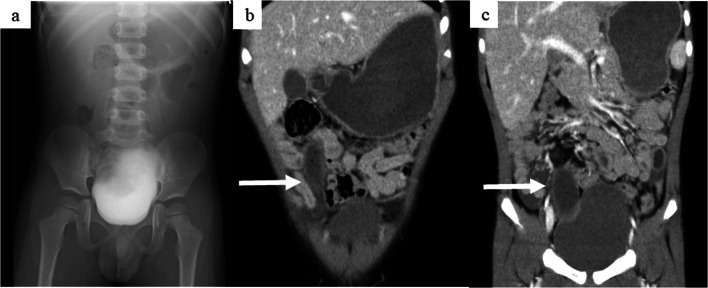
Abdominal X-ray and contrast-enhanced CT. a An abdominal X-ray did not show dilation of intestine and findings of ileus. b, c From the right middle abdomen to the bottom of the pelvis, we detected a gastrointestinal-like lesion (arrow) that was blind with fluid retention inside
